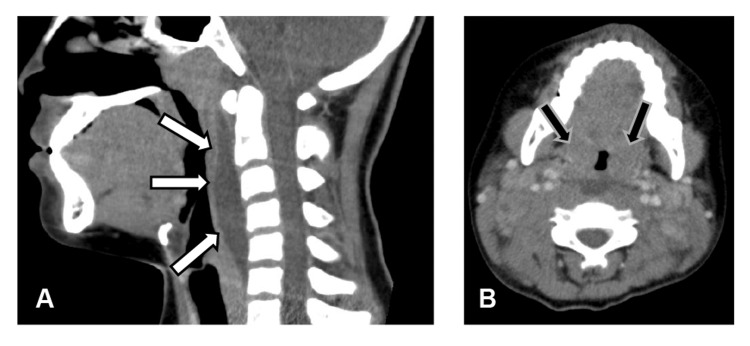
Contrast-enhanced CT scan of the neck of the patient (A) An irregularly shaped low-attenuation area (white arrow) was observed in the pharyngeal space, with no surrounding contrast enhancement. This finding is consistent with retropharyngeal edema, which can be seen in patients with Kawasaki disease. (B) Bilaterally enlarged cervical lymph nodes and hypertrophy of the palatine tonsils are observed (black arrows).
